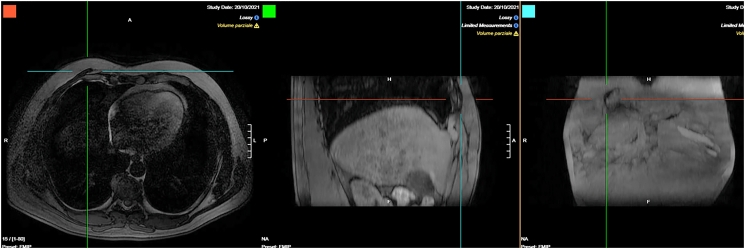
A,B,C. Postoperative thoracic MRI with no evidence of DF local recurrence (panel A axial view, panel B sagittal view, panel C coronal view).
